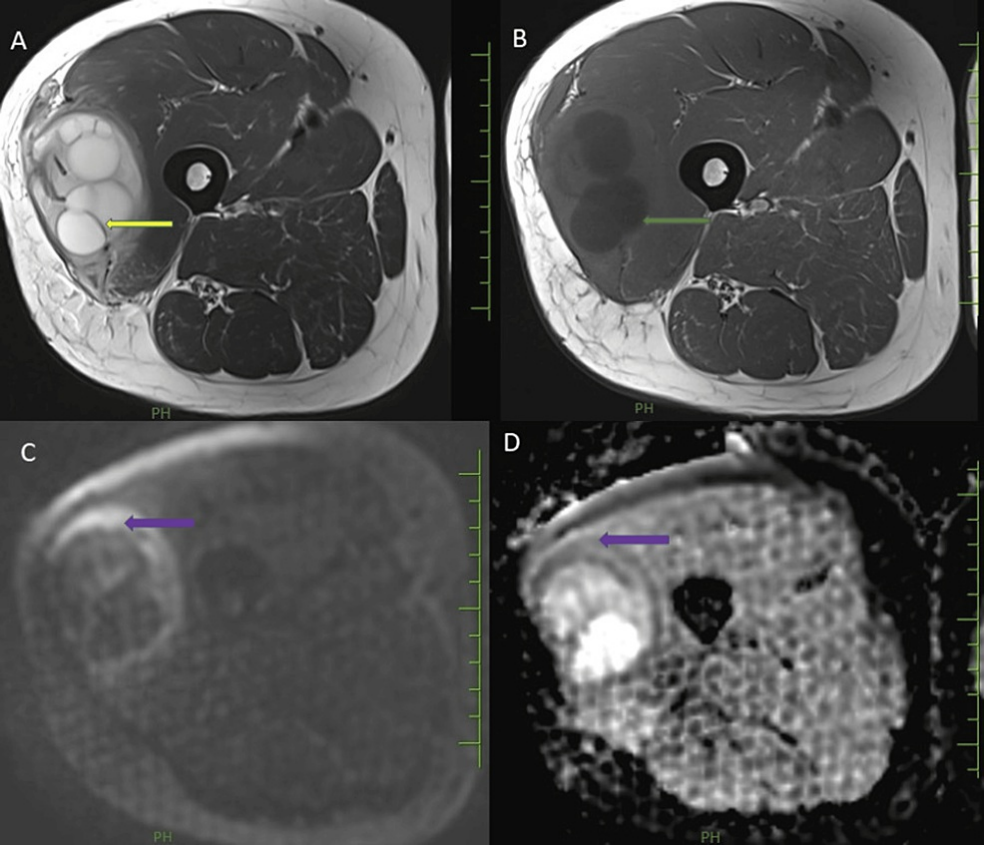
Axial MRI scans of the right thigh showing hydatic cysts. Multiple T2 hyperintense daughter cysts (A, yellow arrow), exhibiting hypointense signal intensity on T1-weighted images (B, green arrow). Diffusion restriction with a corresponding low ADC value was noted in the wall of the cyst (C and D, purple arrow). MRI, magnetic resonance imaging; ADC, apparent diffusion coefficient
